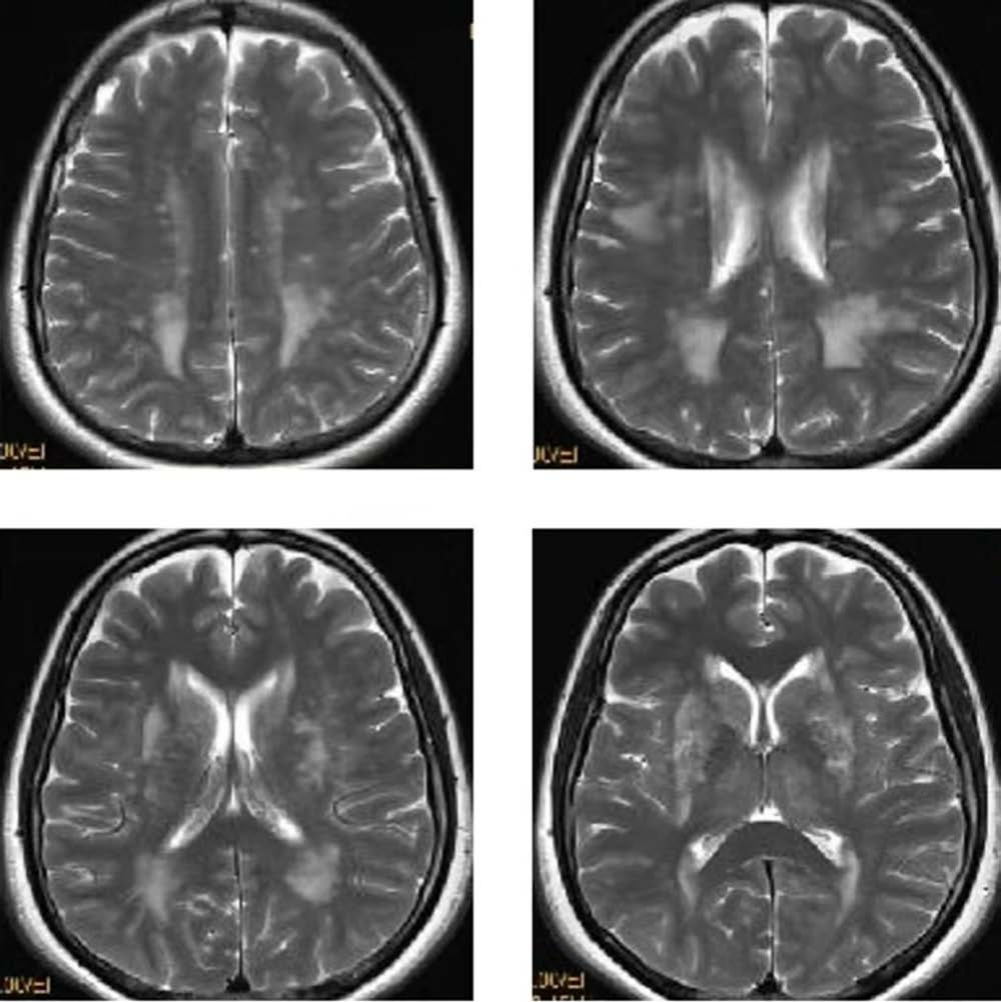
Cerebral MRI images of the patient’s brain. MRI = magnetic resonance imaging.
